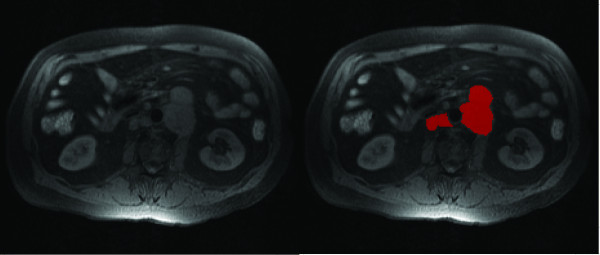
Axial T1-weighted fat saturation image slice of the abdomen of a typical subject (left), and ROI drawn on lymphoma mass (right).
